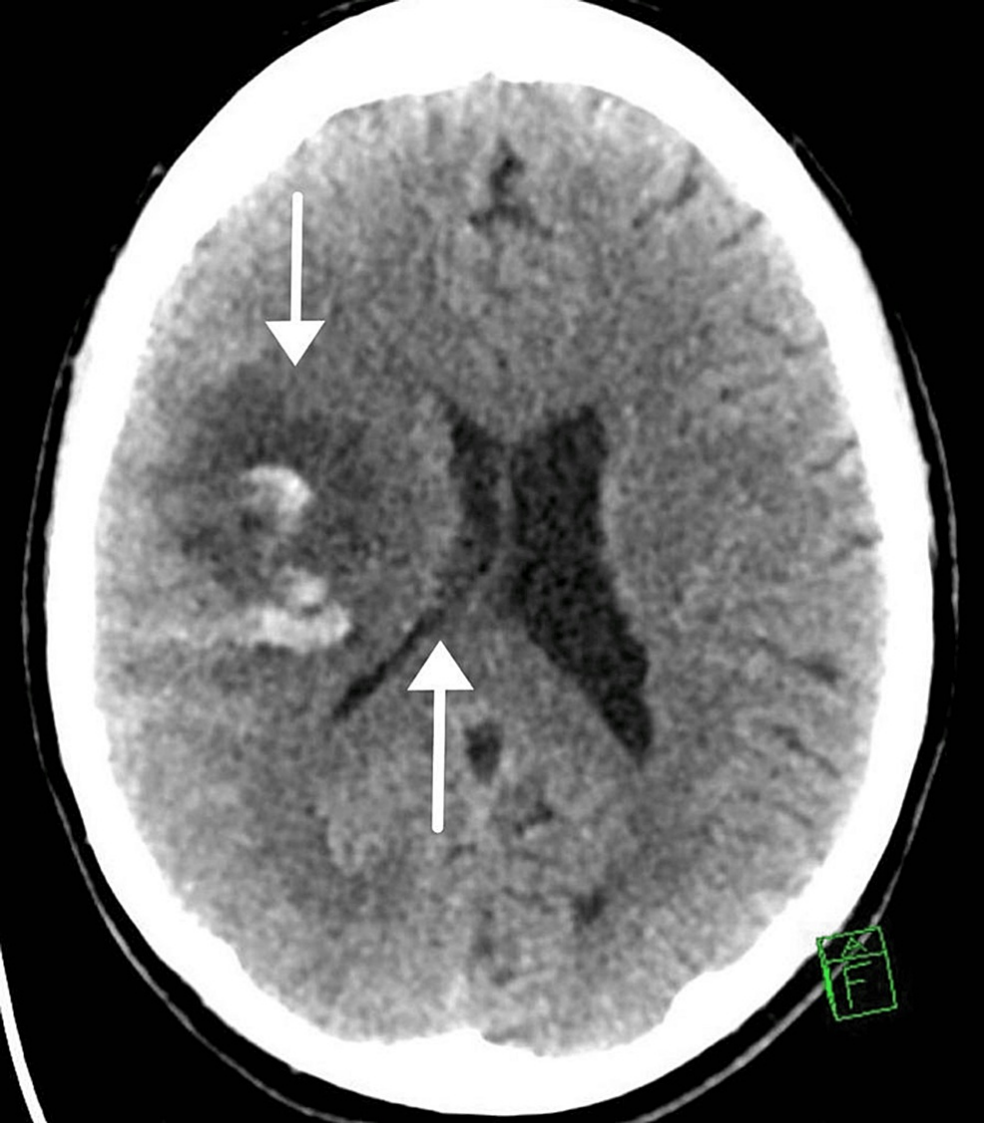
Computed tomography scan of the brain Right middle cerebral artery territory; hemorrhagic infarction associated with mass effect and midline shift
